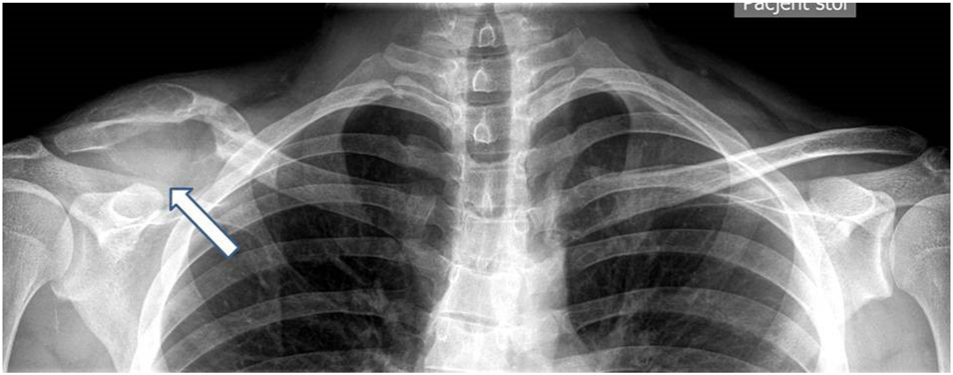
Case 1: x-ray of osteolytic tumor of the right clavicle (arrow)
